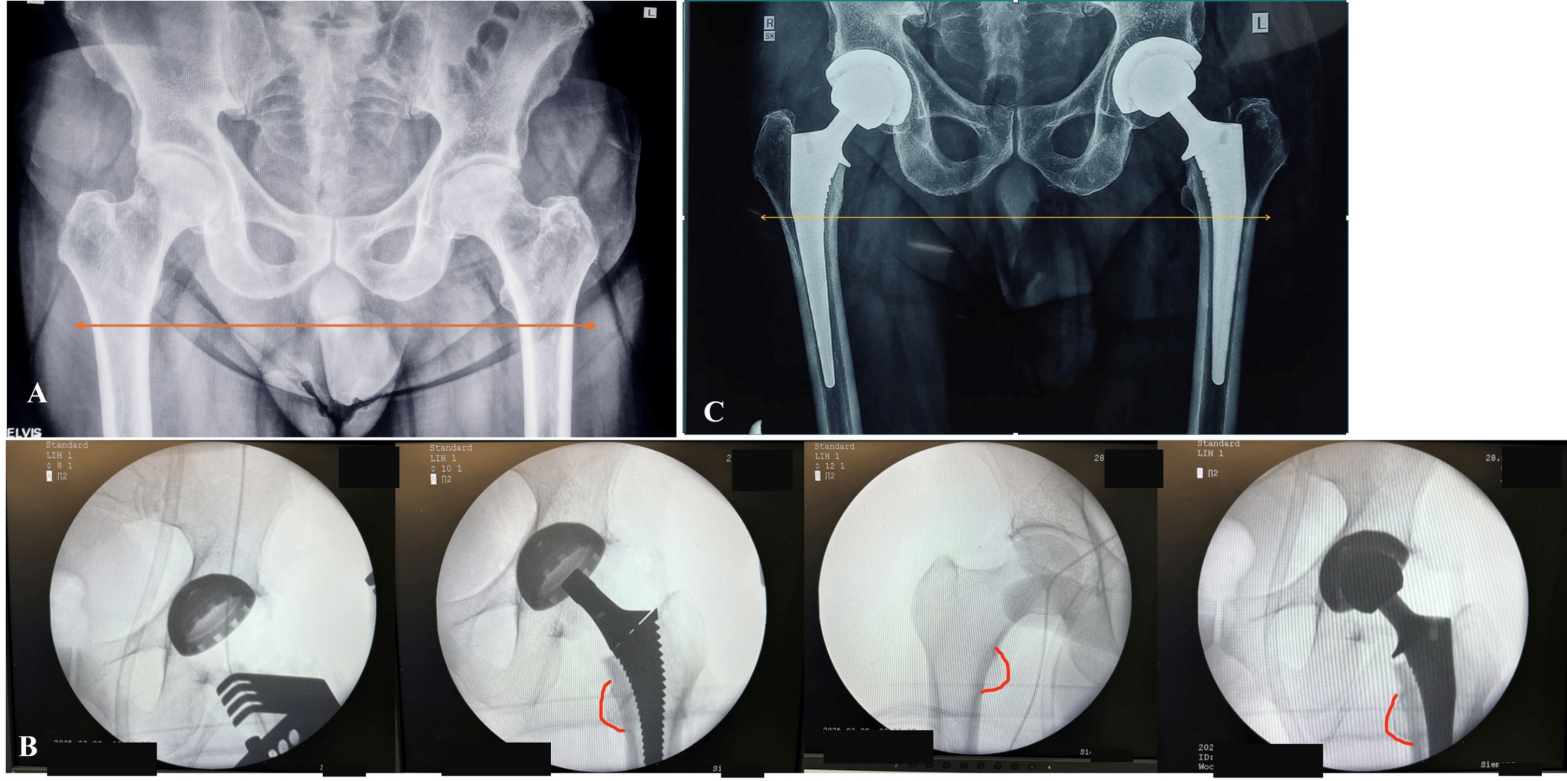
Radiographic assessment and intraoperative technique in direct anterior approach bilateral total hip arthroplasty (THA). Panel A: Preoperative radiograph of a 63-year-old patient with bilateral avascular necrosis (AVN) of the femoral head. Neither the patient nor the surgeon identified any actual or perceived limb length discrepancy (orange arrow). The patient underwent single-stage bilateral THA. Panel B: Intraoperative fluoroscopic images during left THA, utilizing the lesser trochanter (red curved lines) as an anatomical landmark for limb length assessment during a single-stage bilateral THA. Panel C: Immediate postoperative radiograph demonstrating the achieved leg length equality, confirming the accuracy of intraoperative limb length restoration (yellow arrow).
